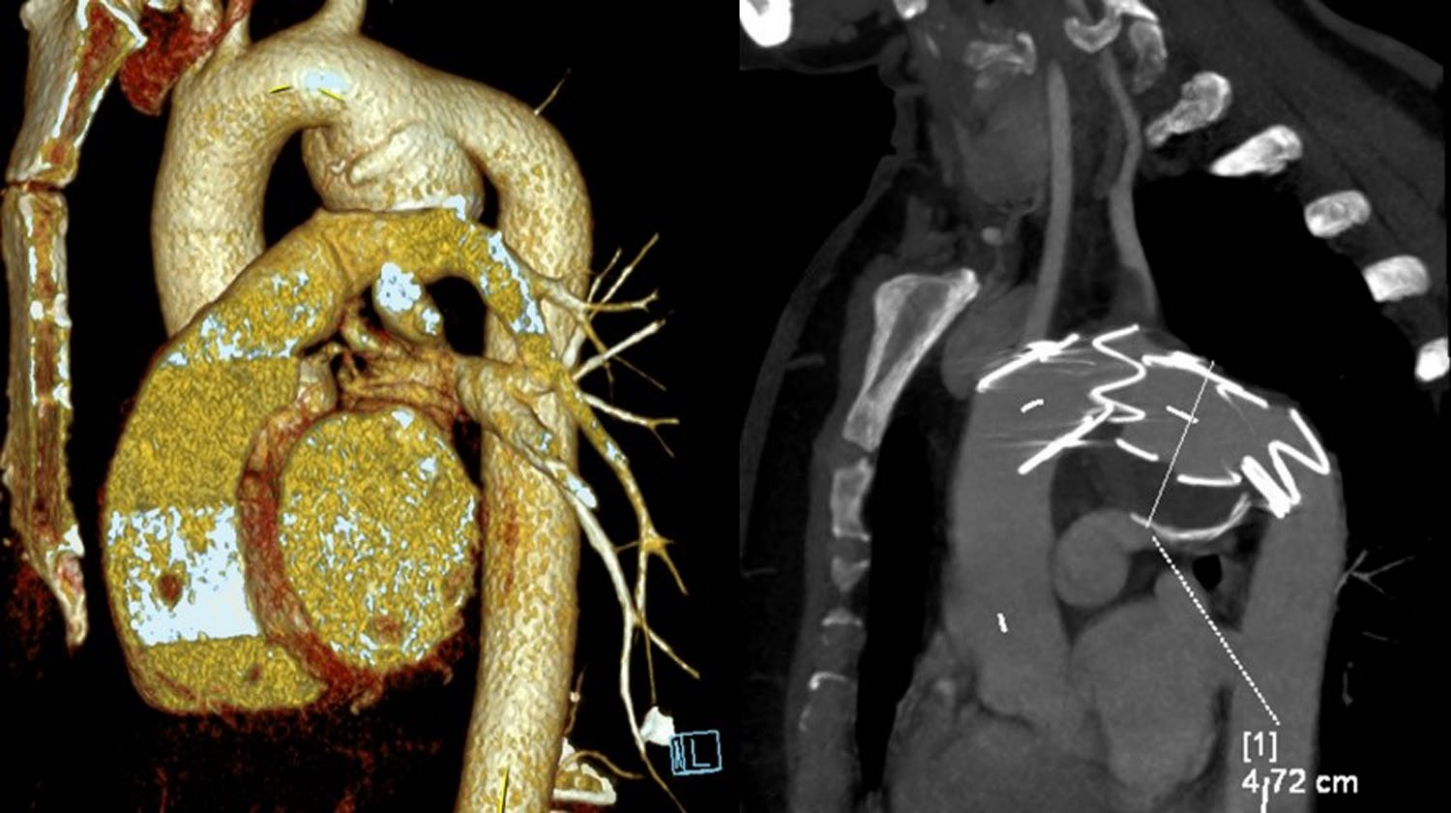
CT scan of aortic pseudoaneurysm caused by trauma and after stentgraft implantation
